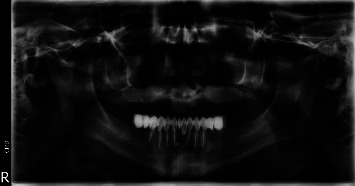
The panoramic X-ray reveals a complete edentulous maxilla with a radiolucent image of the nasal cavity and maxillary sinuses showing nasal destruction with sinus communication.
